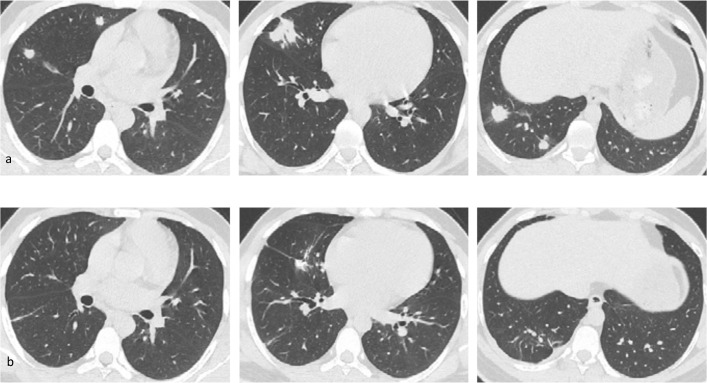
(A) CT scan axial images show multiple parenchymal solid nodules, largest in the right middle lobe. (B) Three months later, control CT images show total regression of the lesions, atelectasis due to the VATS resection.
